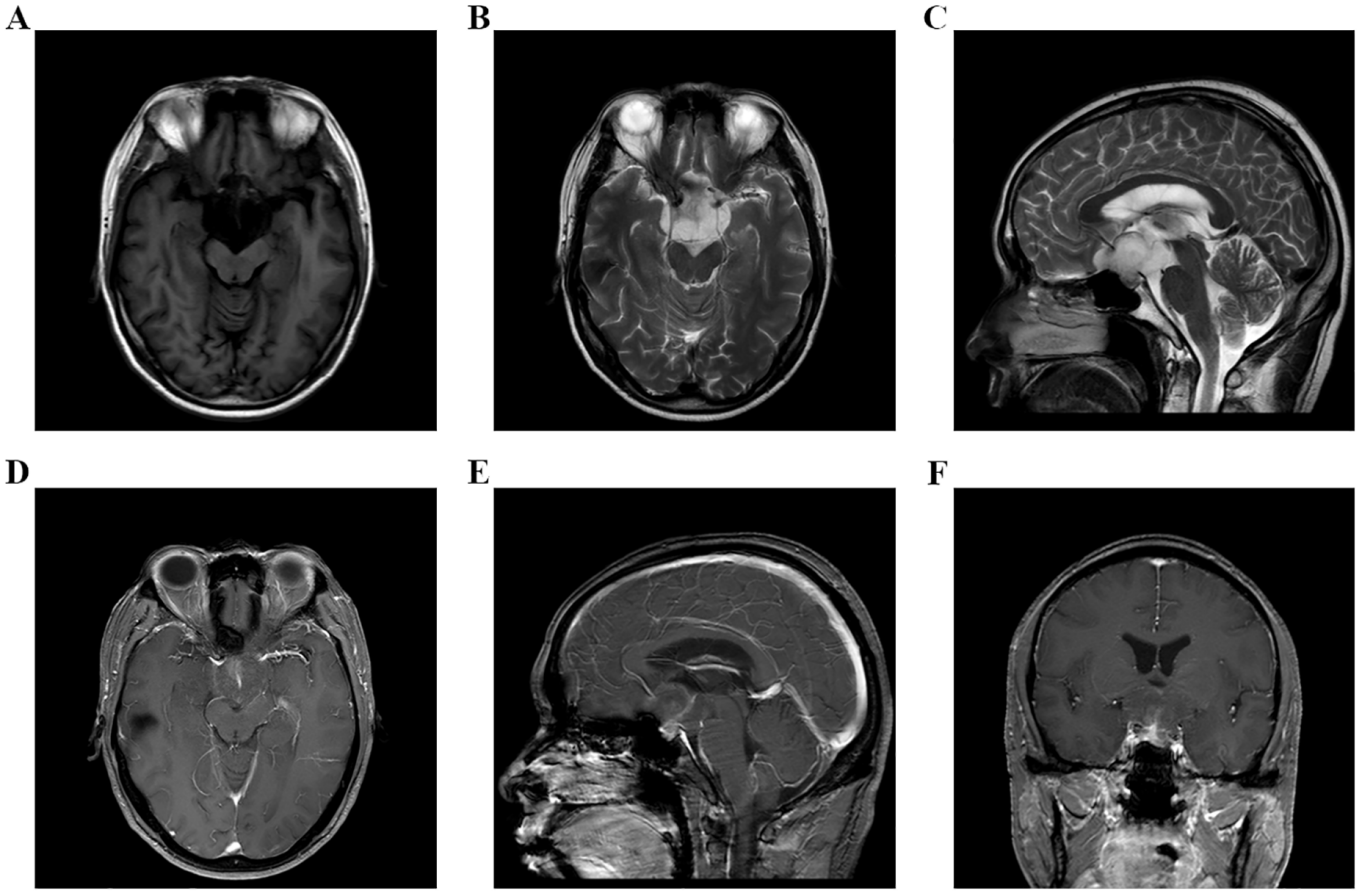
(A–F) MRI examination of head on September 8, 2023, indicated that there was an occupying lesion in the sellar area and suprasellar cisterna, the size was 4.4 × 3.9 × 2.6 cm, and the grade was Knosp3.
